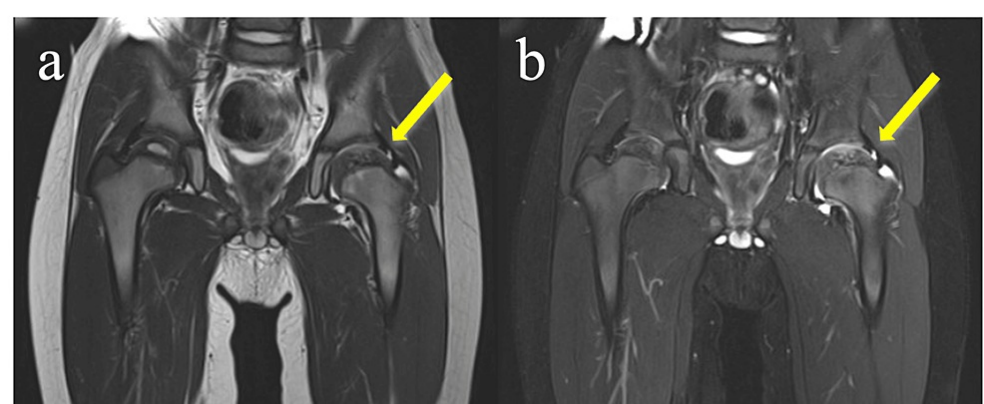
(a) T2-weighted magnetic resonance image of the pelvis showing low intensity of the left femoral head (yellow arrow). (b) Fat-suppressed T2-weighted magnetic resonance image showing high and low mixed intensities of the left femoral head. A little synovial fluid is noticeable (yellow arrow).
